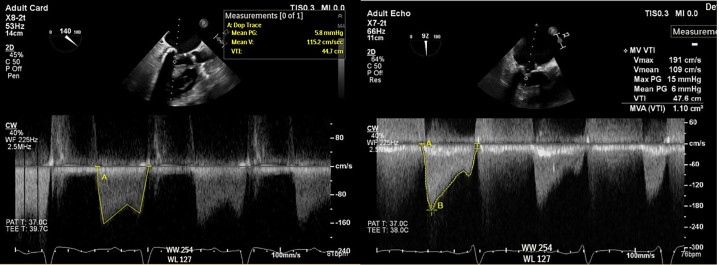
Postprocedural MV Gradients on Transesophageal Echocardiography (A) MV gradient after IVL with improvement to 5.8 mm Hg. (B) Similar gradients after PMBC. IVL = intravascular lithotripsy; MV = mitral valve; PMBC = percutaneous mitral balloon commissurotomy.
